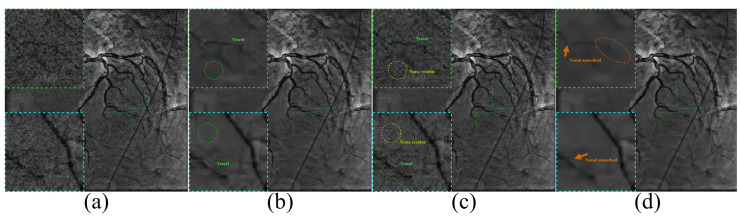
Visual comparison results (a) real-life X-ray angiogram at the 57th frame. (b) Visual result of BM3D with our noise level estimation method (σ=13.0365). (c,d) Visual results of BM3D respectively with empirical noise levels 8 and 18.
